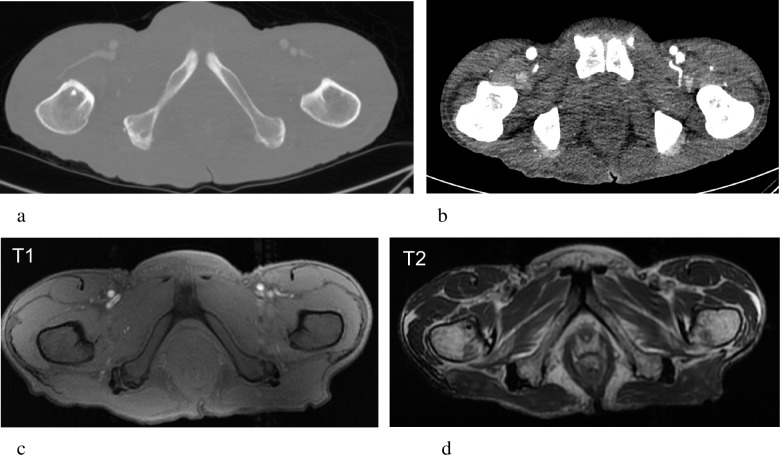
SABM in a 45-year-old male presenting with rapid intentional weight loss, cachexia and deranged liver function on admission. Axial CT image with bone windowing shows normal bone texture, cortical thickness and density (a). Portal-venous phase axial CT image demonstrates a paucity of pelvic fat on the soft tissue windows (b). Diffuse hypointensity of bone marrow on the T1-weighted image (c) and hyperintensity on T2 FS (d) is typical of SABM. Residual visible fat shows abnormal intermediate to hypointense T1 signal and hyperintense fluid sensitive signal. A marked paucity of pelvic fat is also noted
